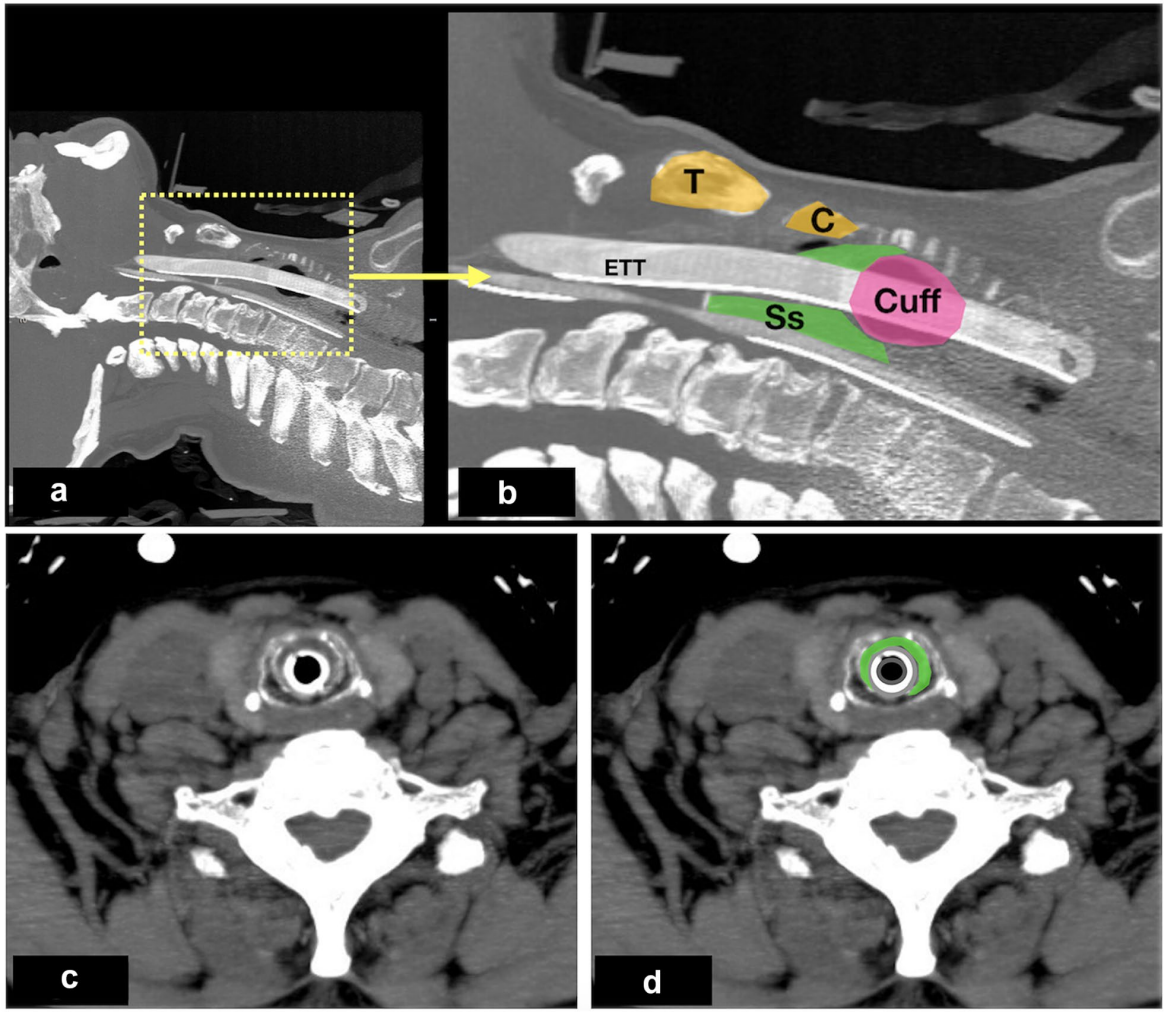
a and b A sagittal view of cervical CT scan showed a subglottic secretions (green watermark) above the ETT cuff (pink watermark) and its relation with other structures; c and d axial views of cervical CT showed subglottic secretions. *Ss* subglottic secretion; *C* cricoid cartilage; *T* thyroid cartilage; *ETT* endotracheal tube
